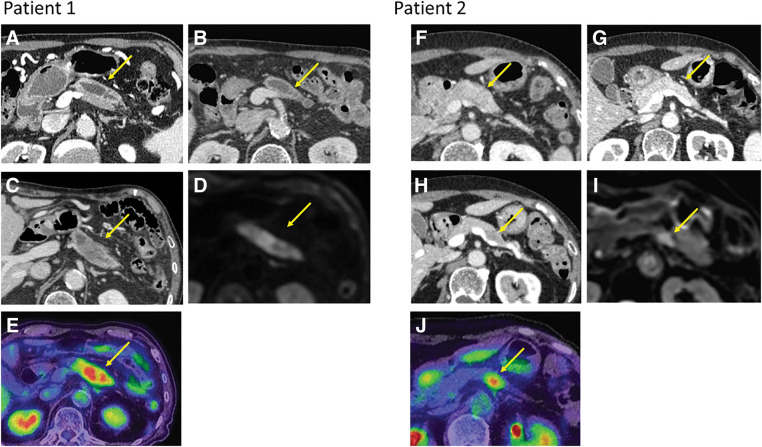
Changes in imaging results of the pancreas over time in two patients. (A, F) Contrast-enhanced CT scan images at AIP diagnosis revealing enlargement of the pancreatic body. (B, G) Contrast-enhanced CT scan images after steroid treatment showing improvement of pancreatic swelling. (C, H) Contrasted-enhanced CT scan images when pancreatic cancer was detected showing a hypodense mass in the pancreatic body. (D, I) Diffusion-weighted MRI revealing a high signal in the pancreatic body. (E, J) PET-CT revealed high uptake of fluorodeoxyglucose in the pancreatic body.
